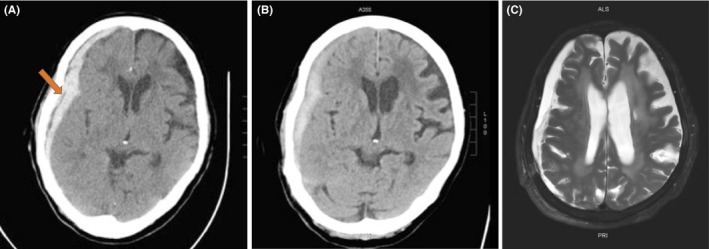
A, Traumatic subdural hematoma on the right temporal area of the patient was confirmed using brain computed tomography (CT) (arrow). B, Brain CT on the 11th day of admission, which was performed just before surgery. There was no significant difference in the hematoma volume observed immediately after admission. C, Brain magnetic resonance imaging (MRI) performed before discharge. Hypoxia‐induced encephalopathy was found along with hydrocephalus
